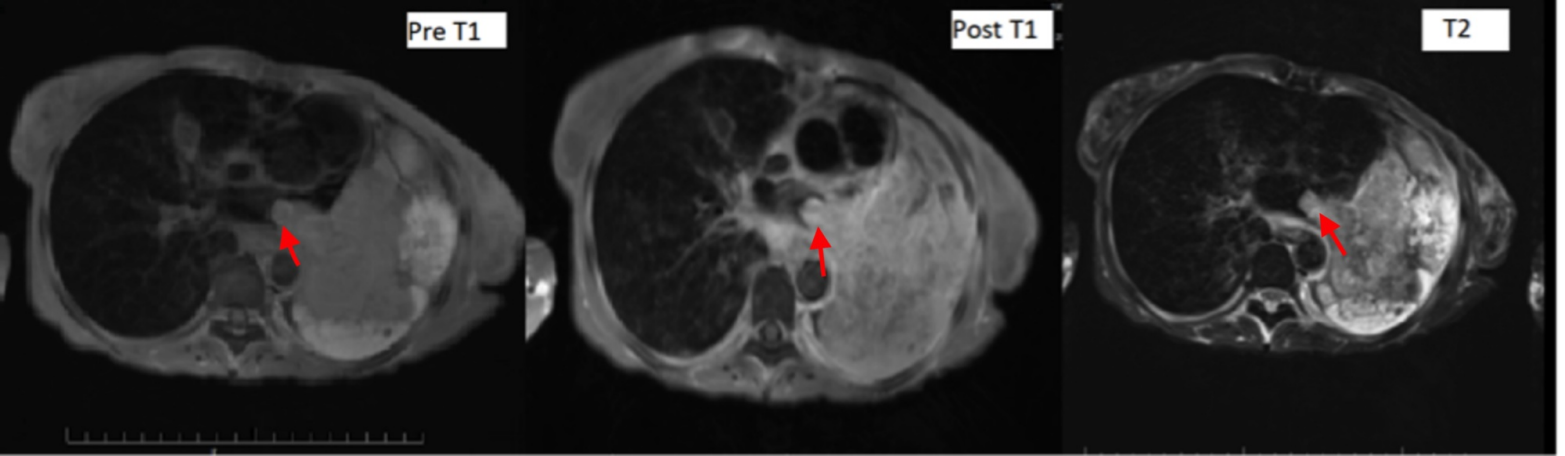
MRI of the heart showing tumor invasion to the left atrium
